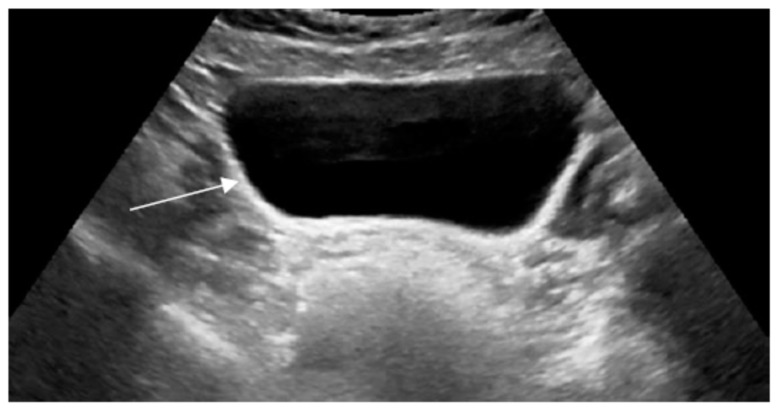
Ultrasound examination scan of the lower abdomen and pelvis. Imaging assessment within the first month of life demonstrated the absence of the uterus and ovaries (not visible below the urinary bladder). White arrow indicates the urinary bladder.
